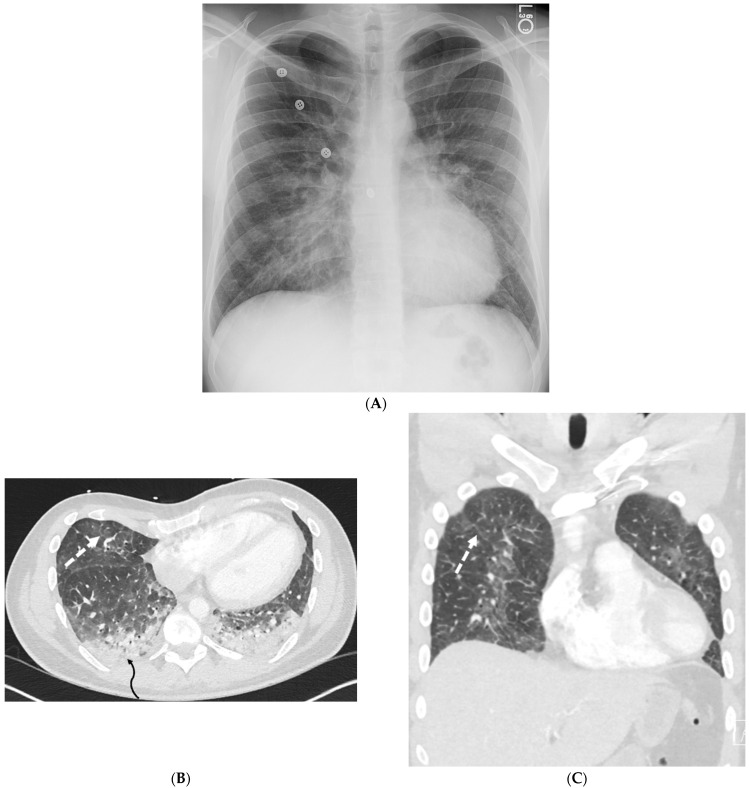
A 25-year-old male with no significant past medical history admitted with acute hypoxic respiratory failure with cough, nausea, and vomiting after vaping one month ago. A frontal chest radiograph (A) and axial and coronal chest CT images (B,C) show diffuse ground glass opacities in the lungs and dependent consolidation (curved black arrow) in bilateral lower lobes. Smooth interlobular septal thickening (dashed white arrow), predominantly in lower lobes, is also visualized on CT images.
